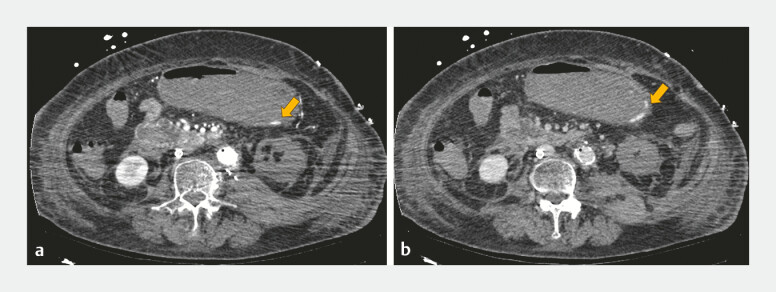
Contrast-enhanced computed tomography showed extravasation of contrast material in the posterior wall of the upper gastric body.
